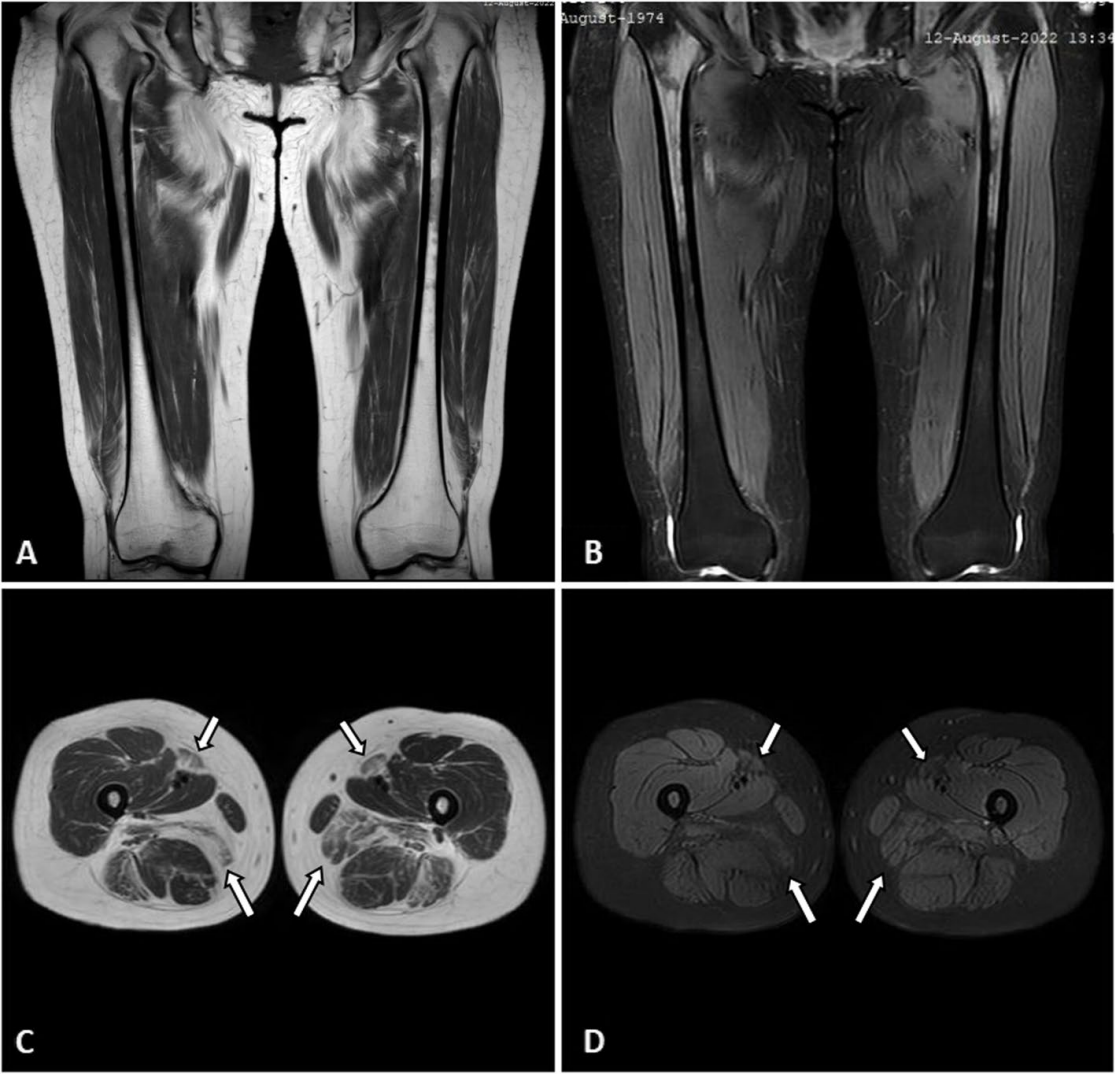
Thigh magnetic resonance imaging of the patient. Magnetic resonance imaging showed bilateral thigh muscle changed consistent with myositis (multiple muscle atrophy and patchy slighted high signal in fat saturated sequence) mainly involved the adductor magnus and sartorius (arrows). A T1-weighted non-fat saturated coronal image; B T2-weighted non-fat saturated coronal image; C synthetic T2-weighted non-fat saturated axial image; D synthetic T2-weighted fat saturated axial image
